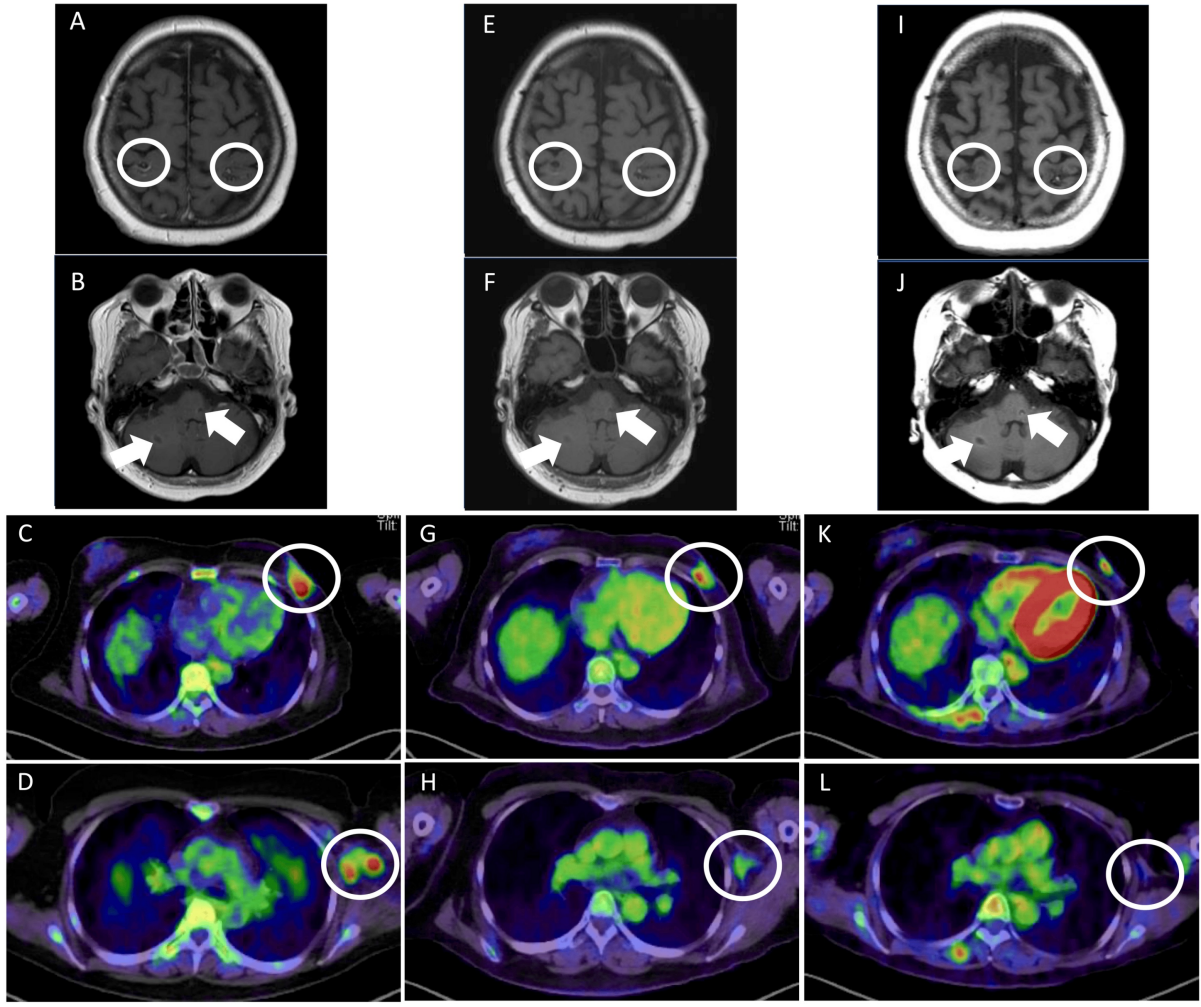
MRI and PET/CT imaging findings Magnetic resonance imaging (MRI) and positron emission tomography (PET)/computed tomography (CT) findings after treatment. (A-D) After treatment with whole-brain radiotherapy (WBRT) and three cycles of epirubicin plus cyclophosphamide (EC), the tumor shrank and disappeared. (E-H) After treatment with bevacizumab and paclitaxel, the therapeutic effect was maintained. (I-L) During treatment with abemaciclib, letrozole, and luteinizing hormone-releasing hormone (LH-RH) agonist, the tumor in the left breast and left axillary lymph nodes continued to reduce, and brain metastases in the cerebrum and cerebellum multiple continued to remain stable. No new lesions were observed.
